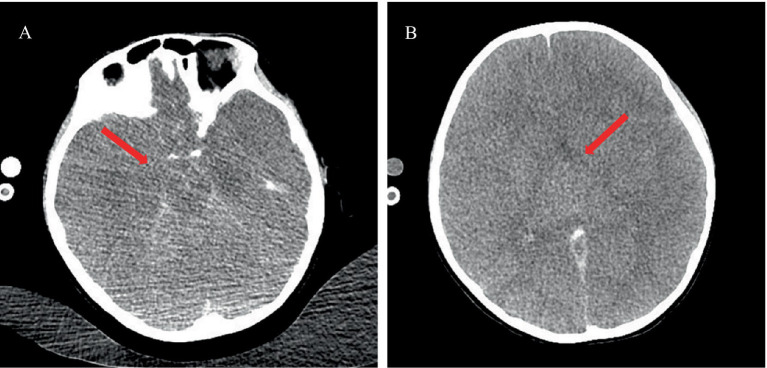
Cranial CT imaging showing herniation. (A) Cranial CT at the level of the cisterns indicating closure of the cisterns (red arrow). (B) Cranial CT at the level of the basal ganglia demonstrating narrowed lateral ventricles, suggestive of herniation (red arrow).
